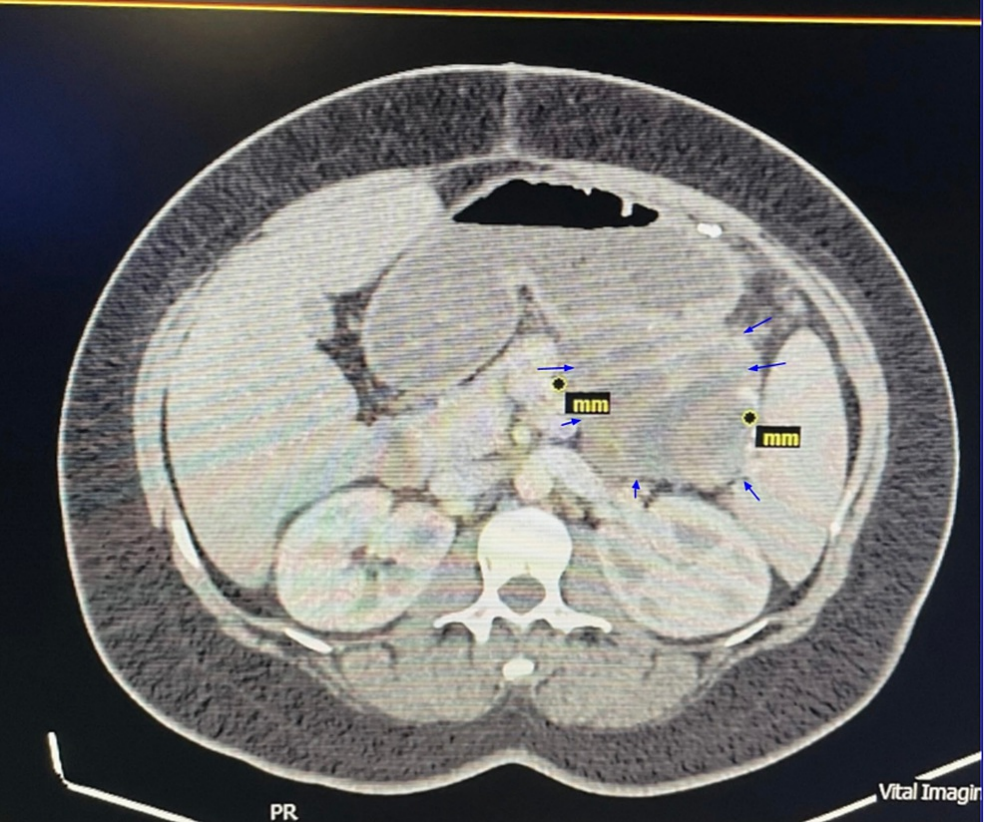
Non-contrast CT scan of the abdomen three months post-surgery. Blue arrows highlight the margins of the pancreatic cyst.
